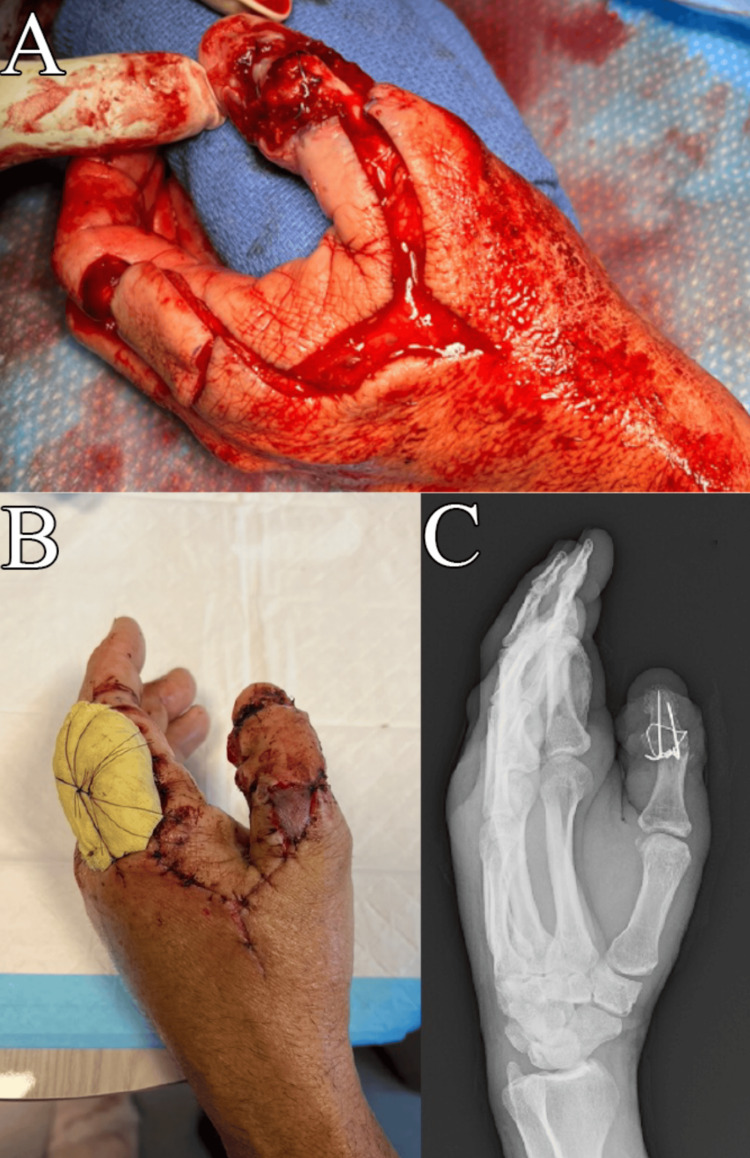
Second-stage reconstructive surgery A: Once margins were confirmed to be negative, a second-stage reconstructive surgery involving thumb interphalangeal joint fusion and FDMA flap coverage was performed; B: Bolster in place over the index finger skin graft site and a viable thumb flap three days post-operatively; C: Radiographic appearance of the fusion construct. FDMA: first dorsal metacarpal artery.
